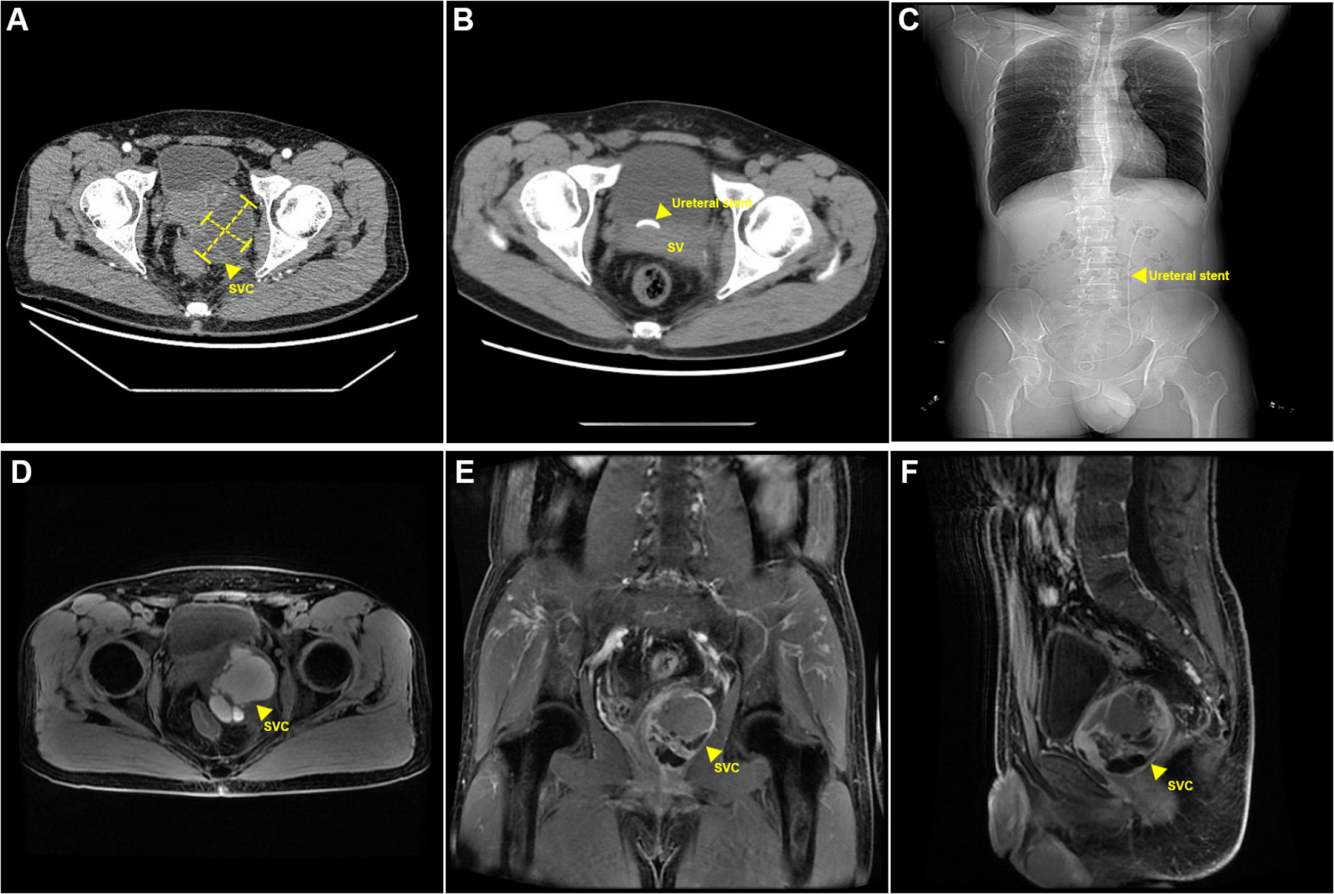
Perioperative radiographic findings. (A) Preoperative CT findings: cystic lesion in the left seminal vesicle. (B,C) Postoperative CT findings: postoperative left ureteral stent placement. (D–F) Postoperative MRI findings: Cystic lesion in the left seminal vesicle with hemorrhage. SVC, seminal vesical cyst.
